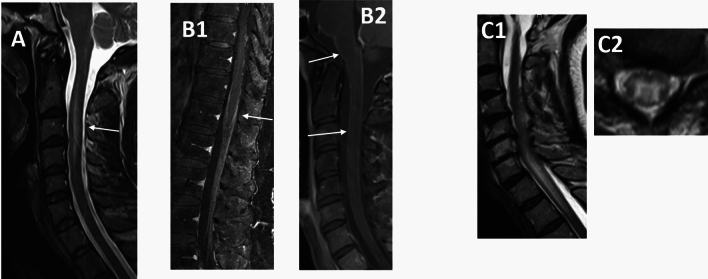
Spinal MRI in LNB patients with spinal affections. (A) longitudinal extensive transverse myelitis (LETM) of the cervical myelon (T2) (B1/2) perimedullar and radicular leptomeningeal enhancement (T1 fat sat with contrast agent) (C) LETM with hyperintense alterations of spinal grey matter (‘H’-sign) in a patient with concomitant CNS vasculitis (T2). White arrows depict representative findings.
